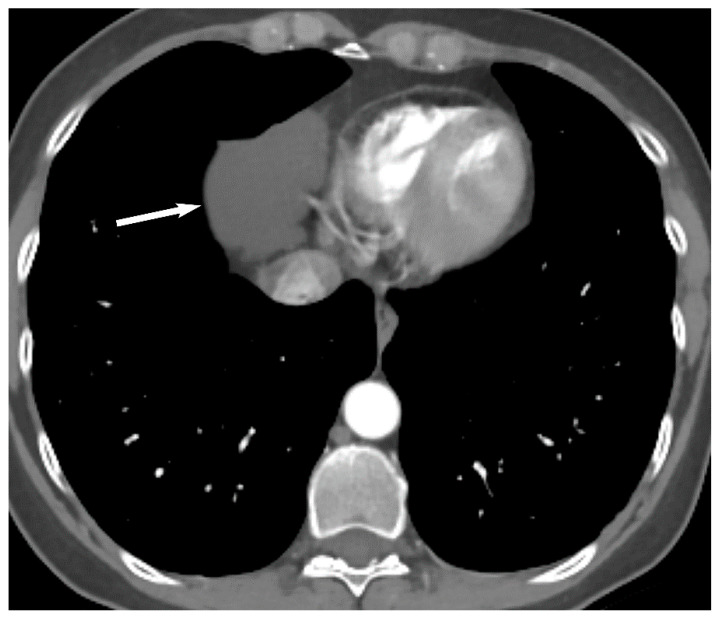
Pericardial cyst. CT shows a fluid attenuating structure (10 Hounsfield units) in the prevascular mediastinum at the right anterior cardiophrenic angle (arrow).
